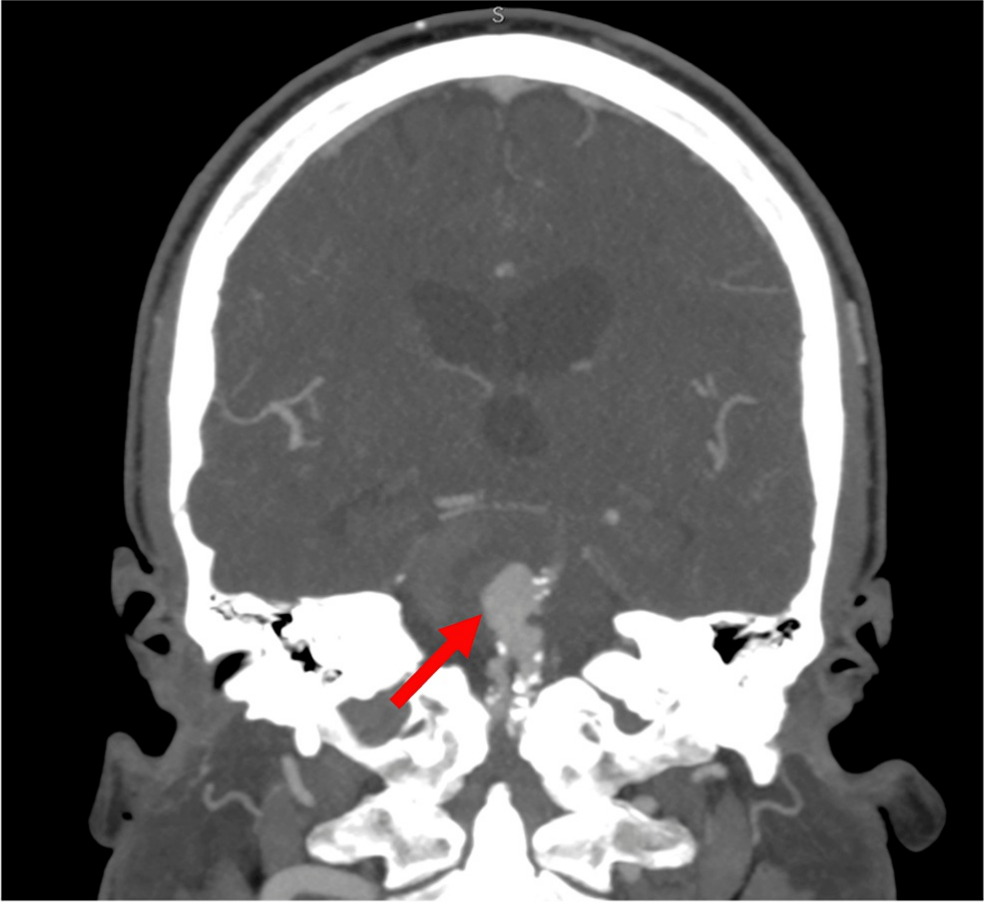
Computed tomography angiography revealing a large, partially thrombosed vertebrobasilar aneurysm (red arrow) with calcified atherosclerotic plaque occupying the distal two-thirds of the basilar artery, causing mass effect on the brainstem and fourth ventricle.
